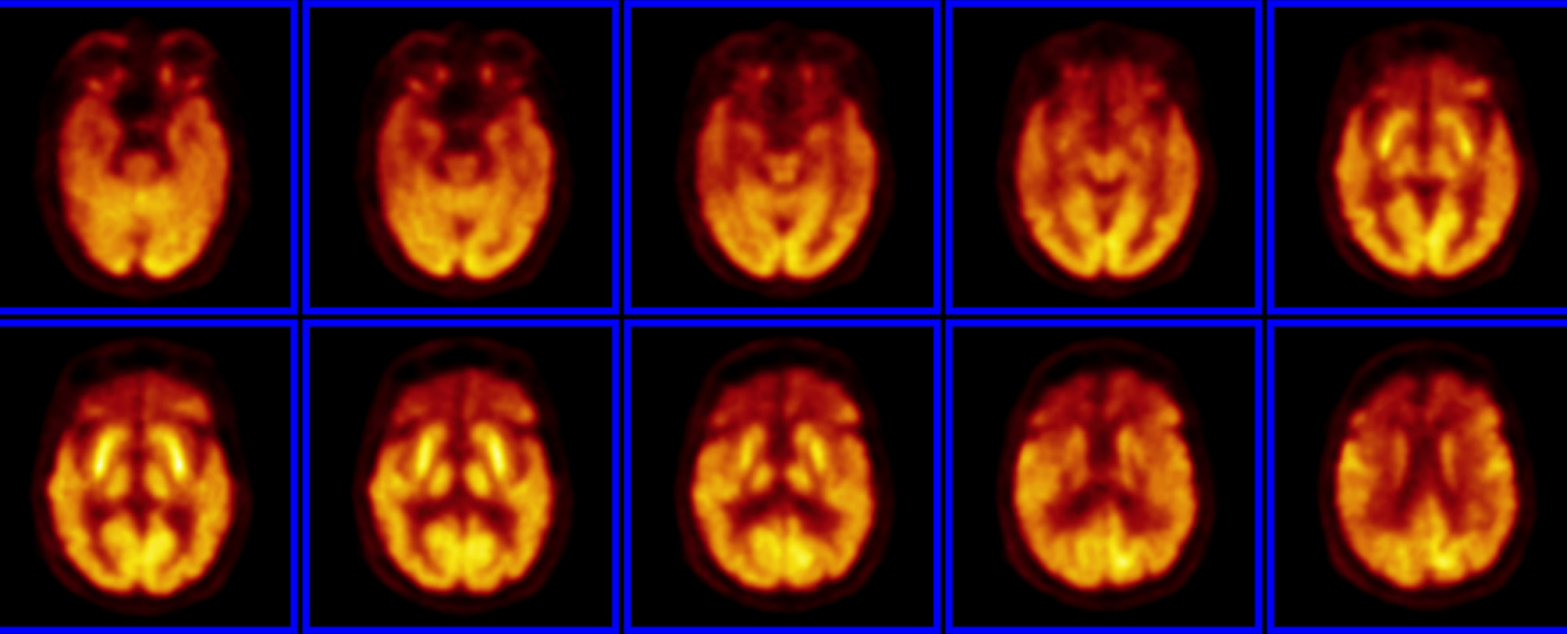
FDG-PET of a patient with a clinical picture of subtle change in behavior
